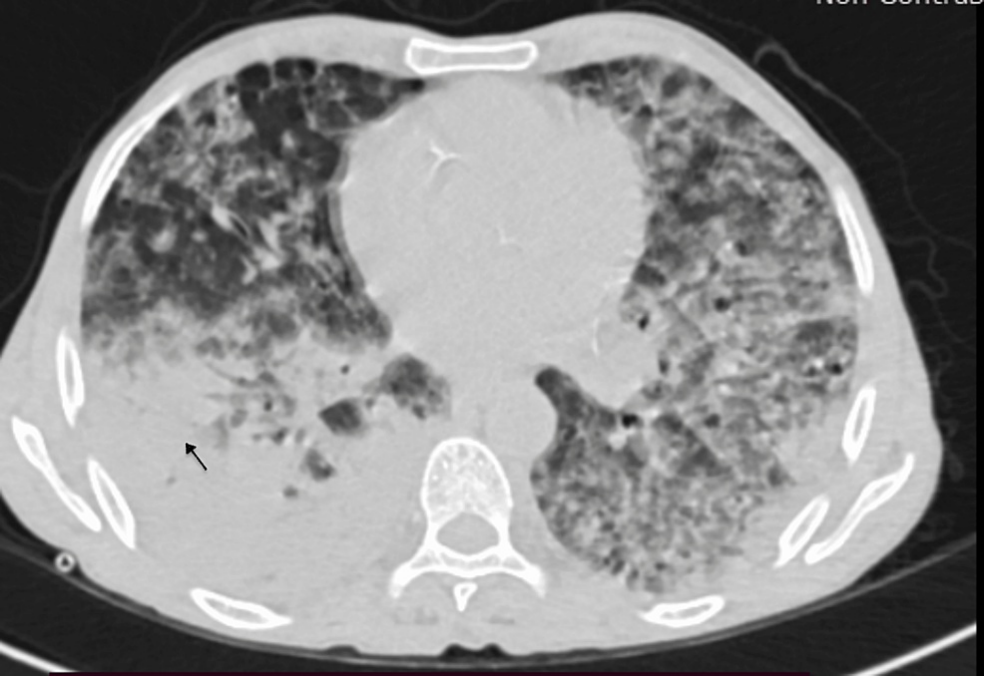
CT thorax showing large confluent areas of consolidation (black arrow), seen involving both the lungs (right lower lobe, left upper and lower lobes) with areas of ground glass opacities in rest of the bilateral lung parenchyma
